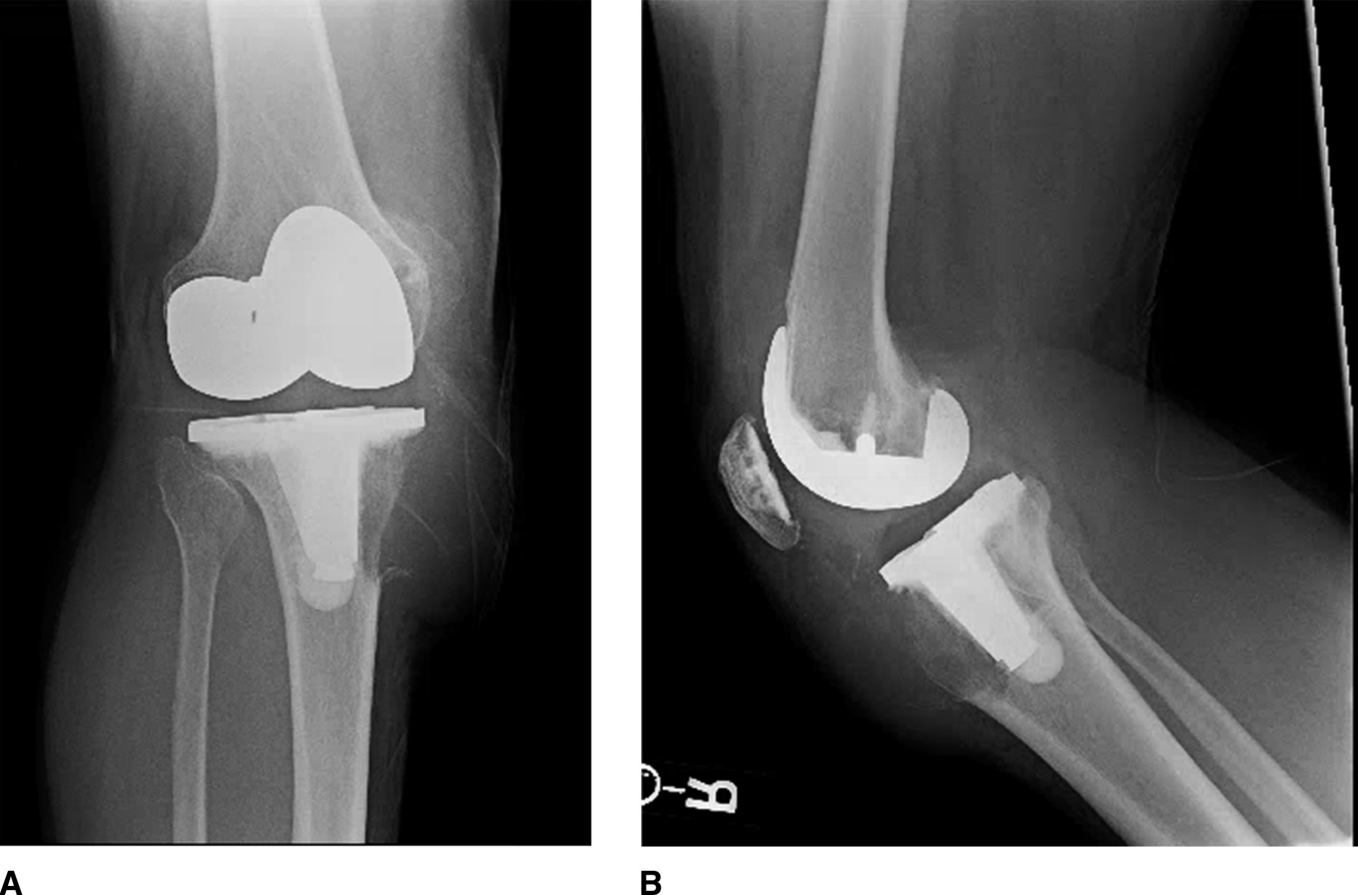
Preoperative radiographs of the right knee demonstrating a lytic juxtaprosthetic lesion of the proximal tibia.
